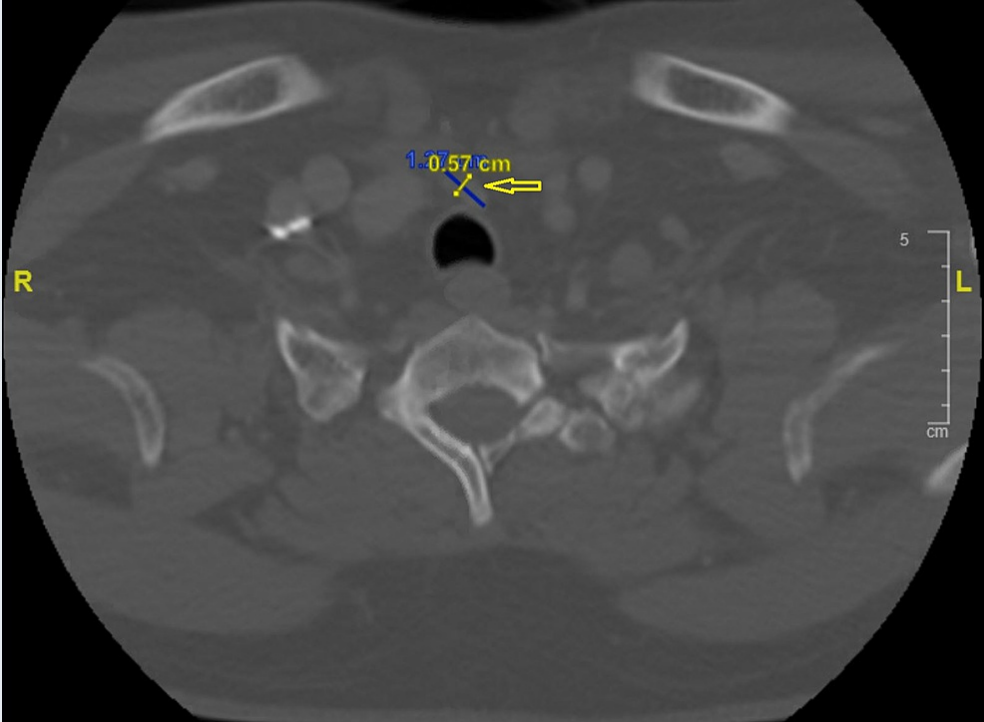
CT neck with contrast showing soft tissue nodule (yellow arrow) measuring 0.57 cm × 1.27 cm in thyroidectomy bed suggestive of tumor recurrence
